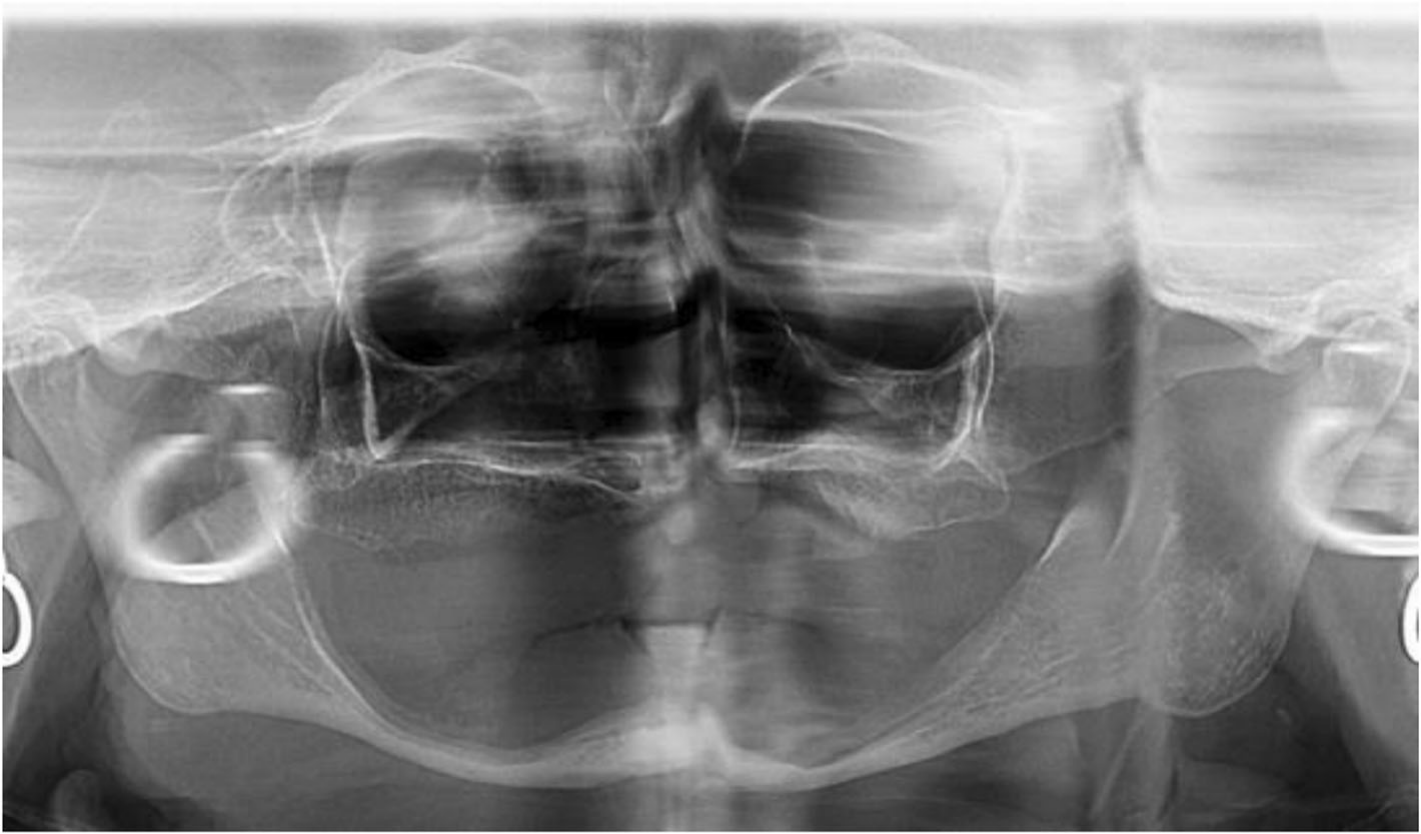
Preoperative orthopantomography
